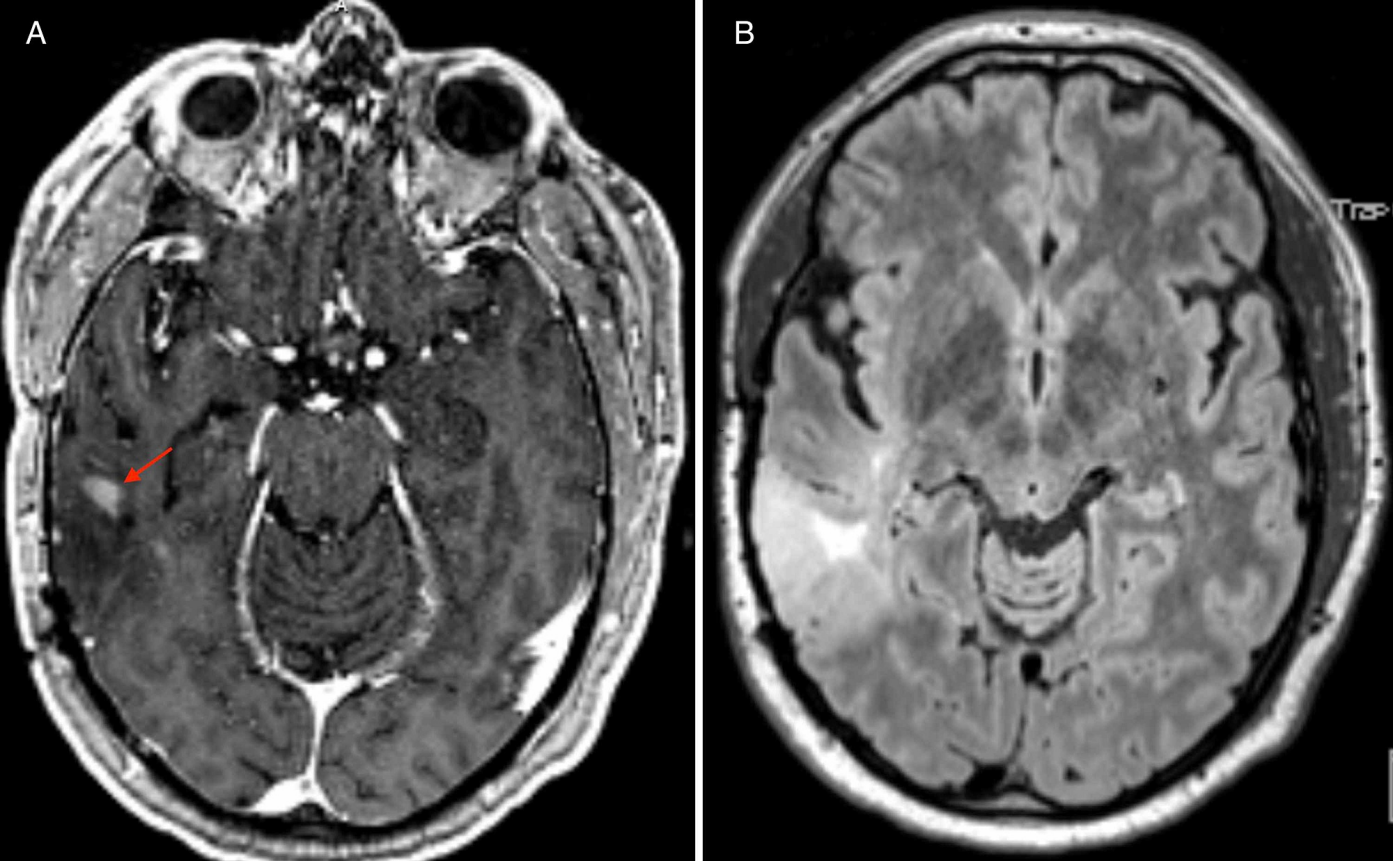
Preoperative T1-weighted MRI with contrast demonstrating an enhancing focus (red arrow) in the right posterior temporal lobe adjacent to the resection cavity for the patient’s prior grade II astrocytoma (A). Preoperative FLAIR MRI demonstrating hyperintensity in the right posterior temporal lobe, which had increased in size from previous studies (B). Pathology obtained during surgery confirmed WHO grade III anaplastic astrocytoma. MRI, magnetic resonance imaging; FLAIR, fluid-attenuated inversion recovery
